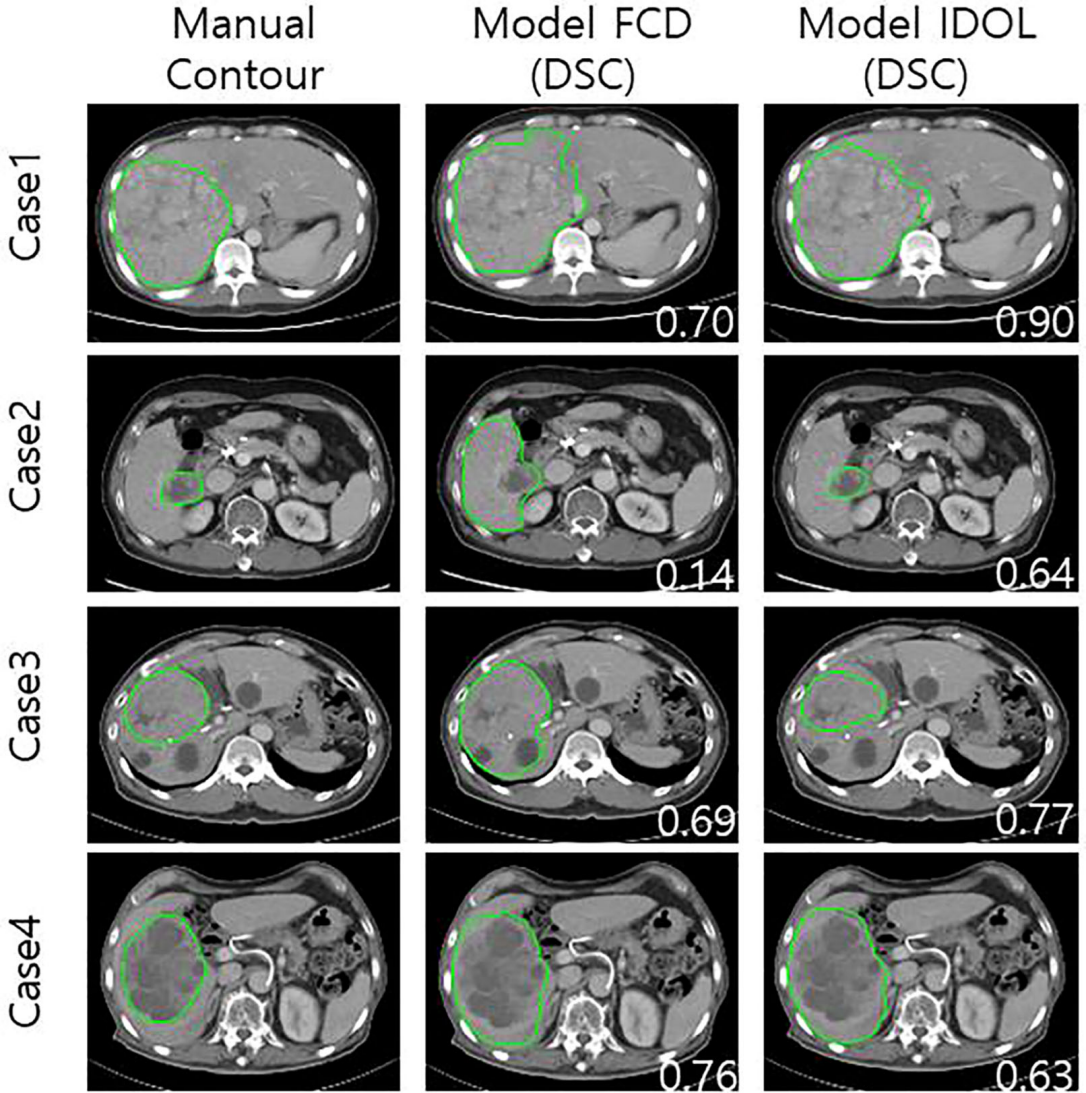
Examples of tumor prediction model and comparison with manually drawn contour in RECIST cohort. Tumor contours on CT scans after treatment are arranged in rows according to manual contour and model FCD & IDOL.
